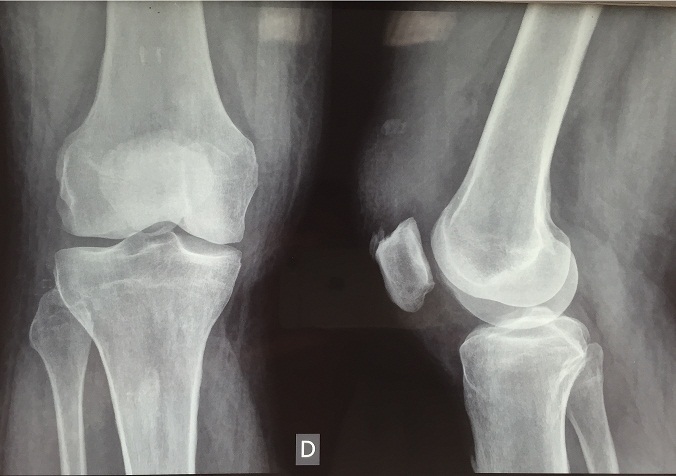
Radiographies standards du genou droit (face+profil) montre une rotule basse.
